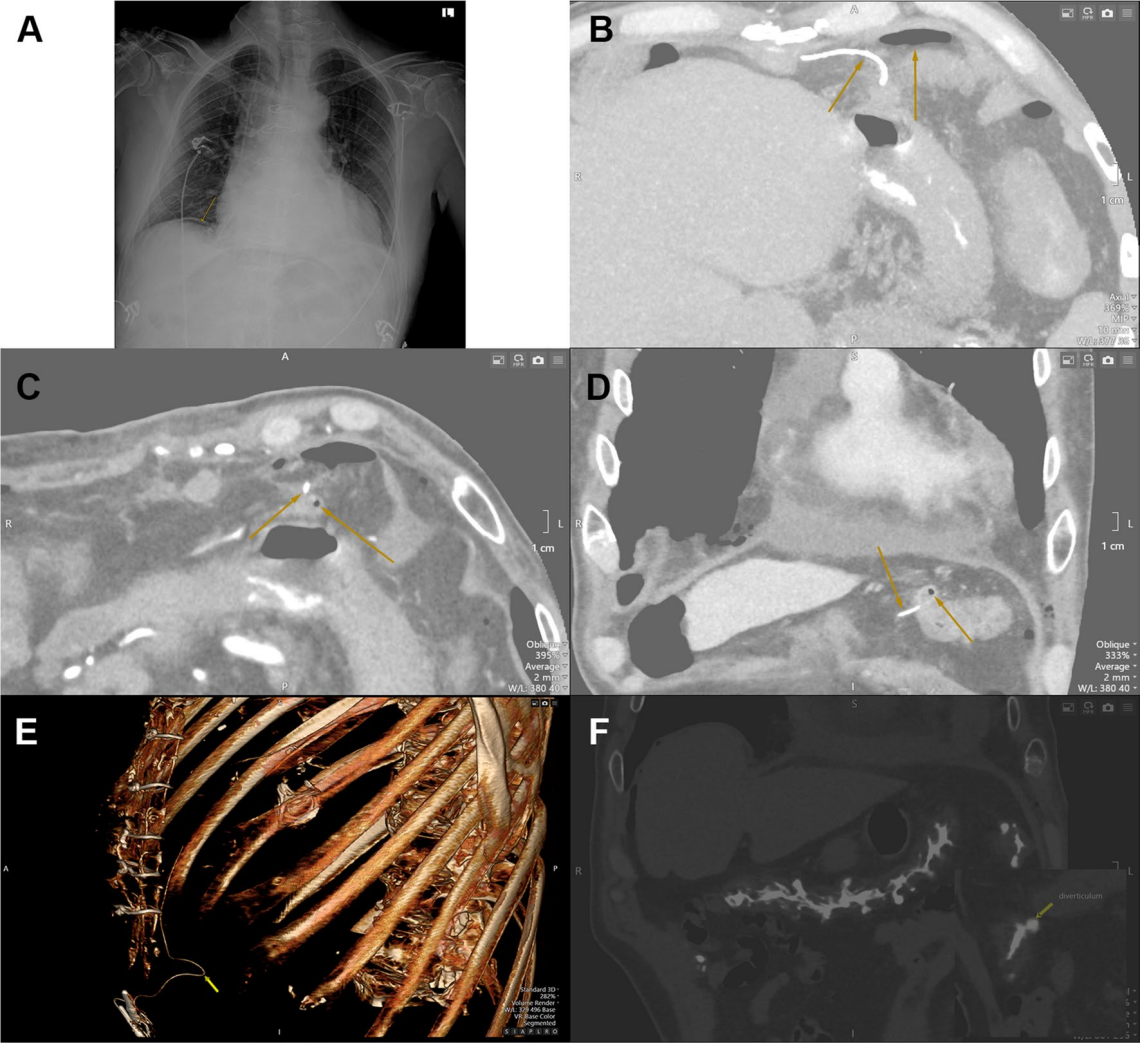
A, B: postoperative chest x-ray and computed tomography showing the pneumo-peritoneum C, D, E: computed tomography showing the injury of the transverse colon at the base of a diverticulum due to the needle end of temporary pacing wires passing through it F computed tomography showing the diverticulum of the transverse colon
